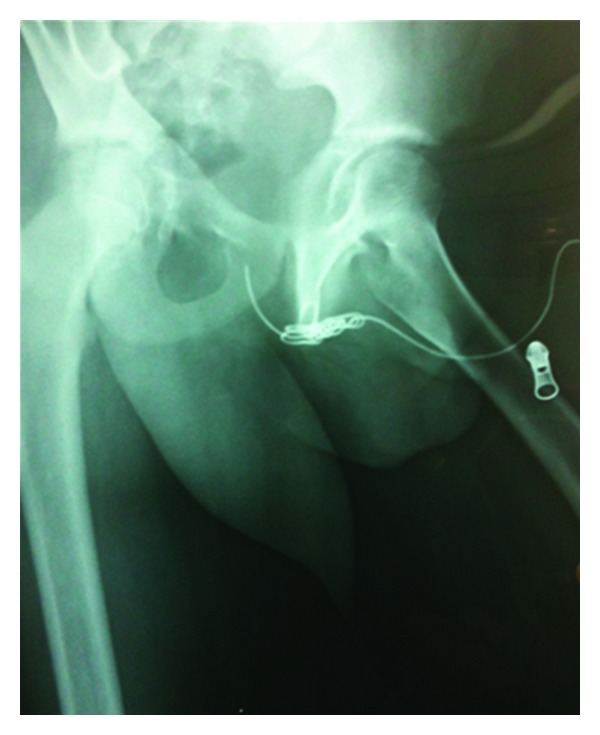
Pelvic plain radiography: twisted cable forming a coiled refractory structure in the posterior urethra.
